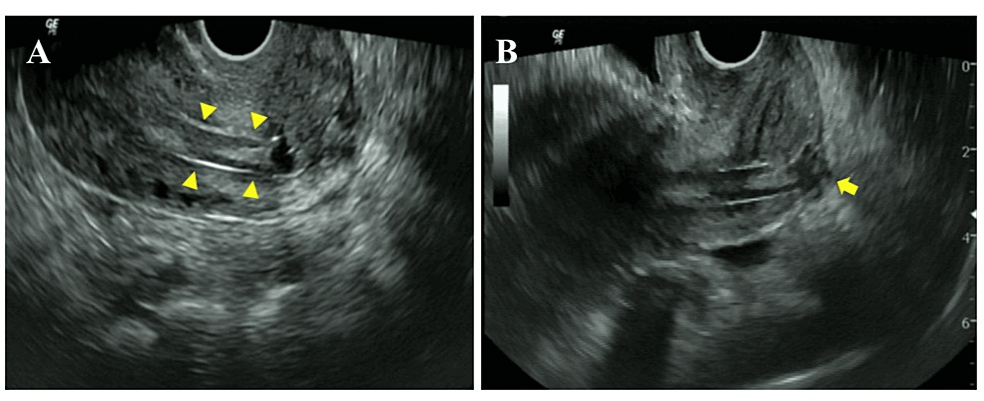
Transvaginal ultrasound images A: Image of artifact in the uterine cavity (arrowheads indicate an artifact in the uterine cavity); B: Image of partial intrusion to the posterior myometrium of the uterus (the arrow indicates a finding suspicious of partial intrusion to the posterior myometrium of the uterus).
